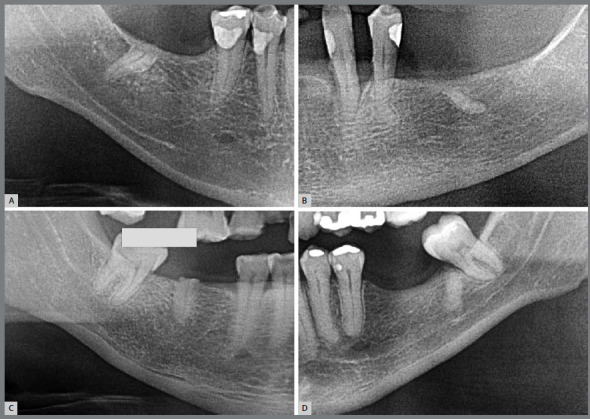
Submerged roots, in the Early Phase of Evolution (A and B) and in the Intermediate Phase (C and D). After the analysis by panoramic radiography, a more detailed tomographic evaluation is suggested, for control and planning of the case.
